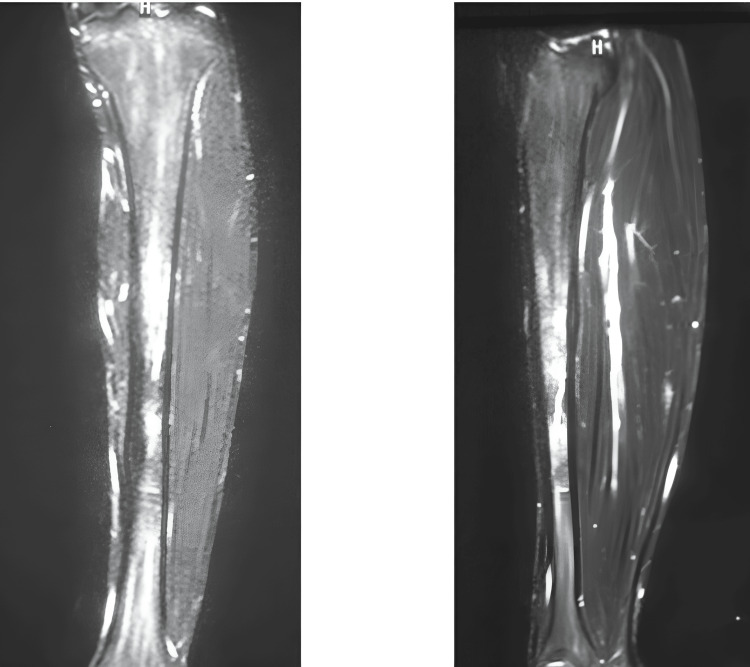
Radiograph of the left lower leg following total fractures of the right leg STIR displays an unusually high signal on imaging. STIR: short tau inversion recovery
